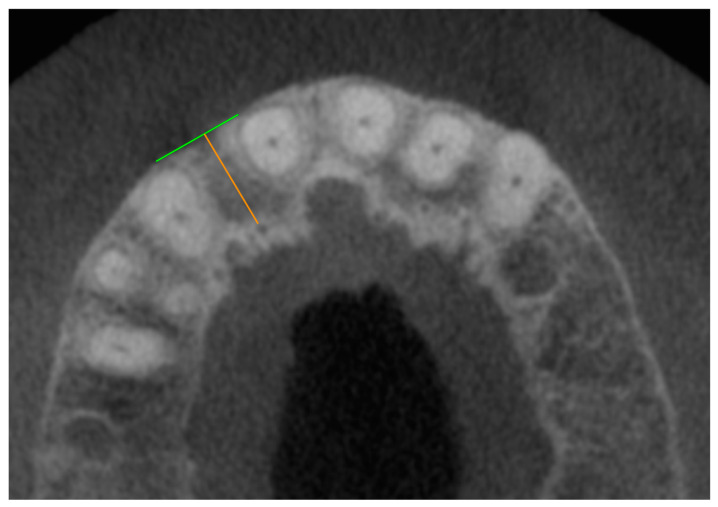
CBCT dataset with the axial representation of an accessory foramen of the canalis sinuosus palatal to missing tooth 12. The green line forms a clearly recognizable outer cortex of the alveolar ridge as an endpoint of the orange measuring section, which begins at the outer edge of the foramen.
